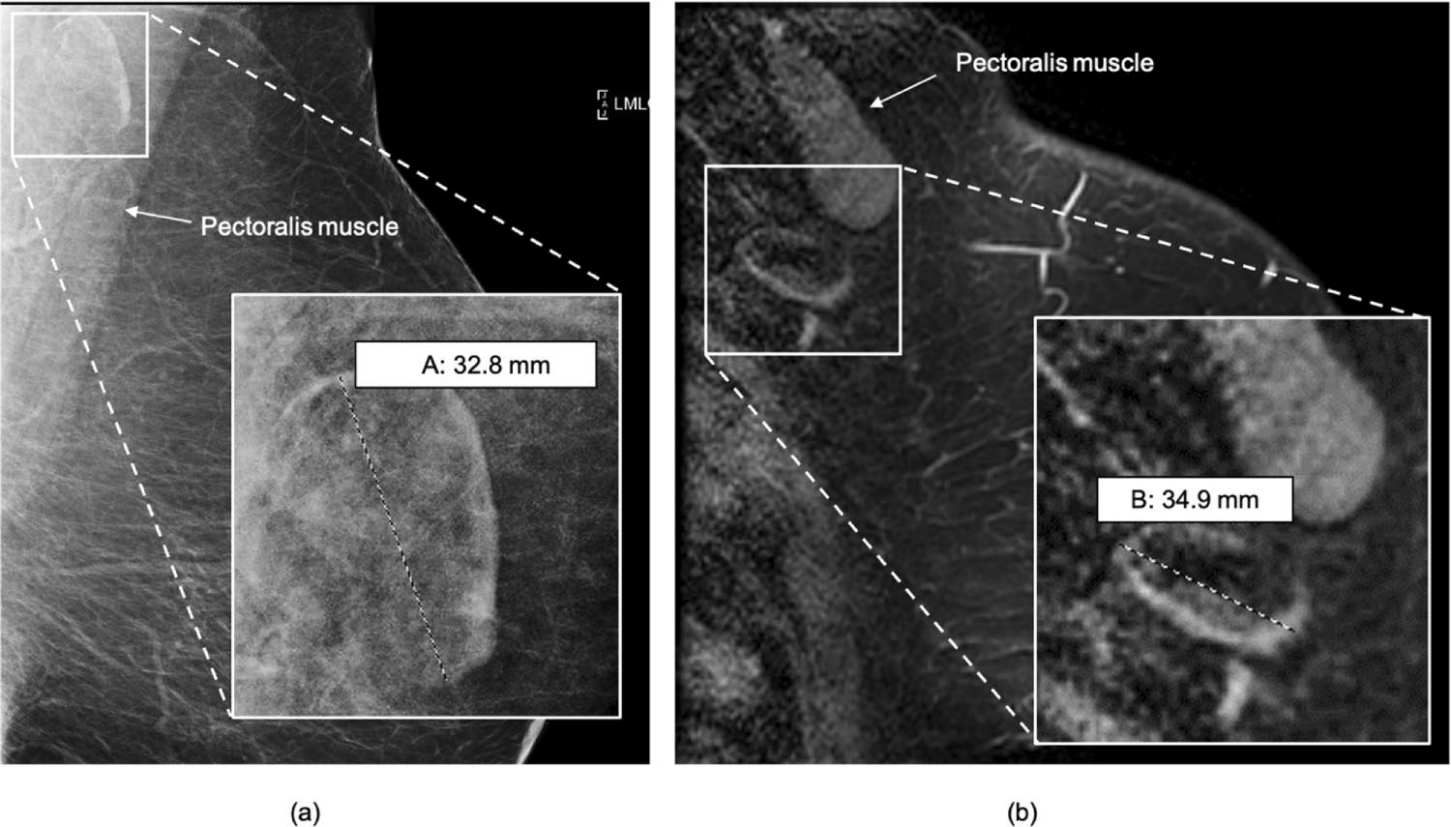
Comparison of LN measurements on mammogram and breast MRI. 54-year-old female with right breast ER+ HER2+ IDC demonstrating contralateral fat-expanded node on mammography compared to breast MRI. a Left MLO digital mammogram shows a fat-expanded lymph node in the contralateral axilla measuring 33 mm in largest dimension, b sagittal fat-saturated enhanced breast MRI of the same patient demonstrates a slightly larger size of the same lymph node measuring 35 mm in length on MRI
